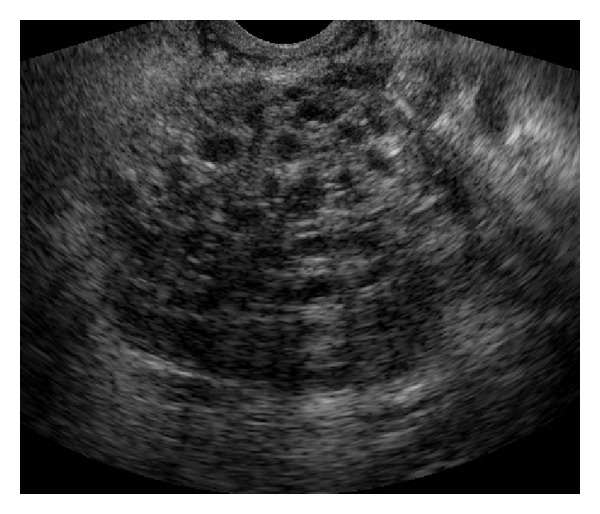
Transrectal ultrasound. Multiloculated solid mass of the right seminal vesicle.
